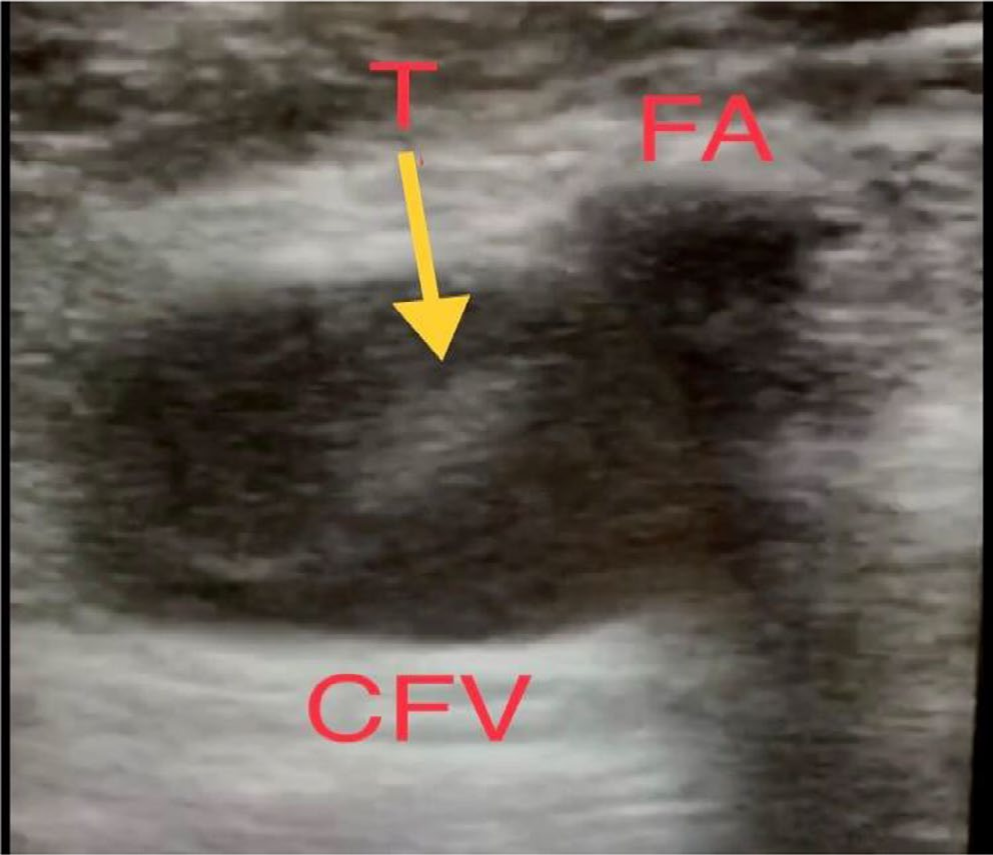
Doppler venous system of noncompressible right common femoral vein partial thrombosis (T). CFV, common femoral vein; FA, femoral artery; T, thrombus
